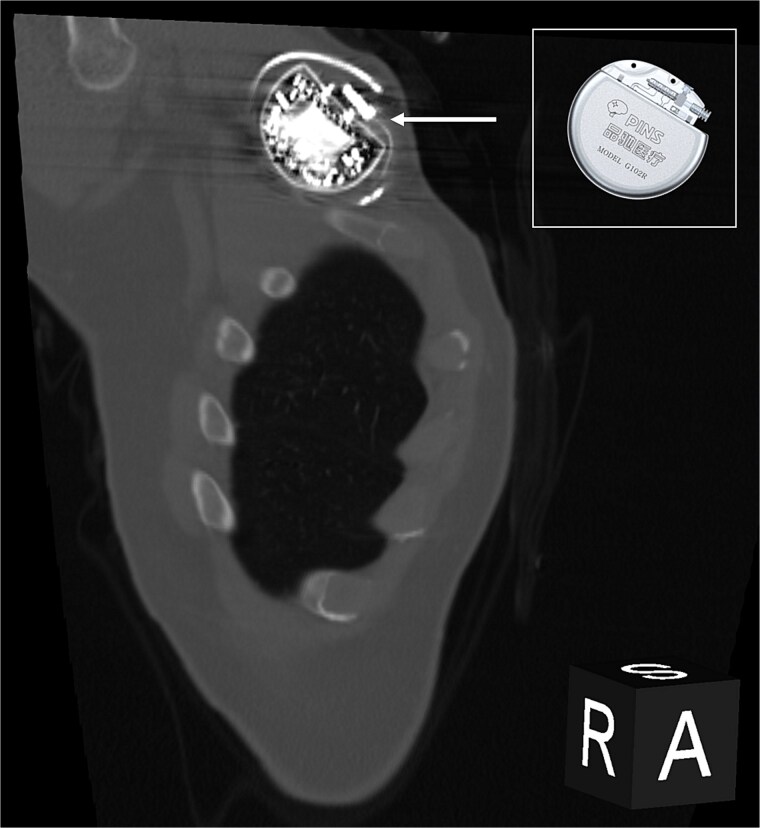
Chest CT performed after the initial deep brain stimulation implantation in 2022 demonstrating appropriate positioning of the rechargeable pulse generator (r-IPG) within the right subcutaneous chest pocket. The anterior, coil-facing surface of the device is oriented towards the overlying skin (upper right inset: Actual anterior view of the generator), representing the normal configuration required for efficient transcutaneous charging. The leads exit the device from the left side (white solid arrow) without coiling or displacement. No rotation or migration is identified.
